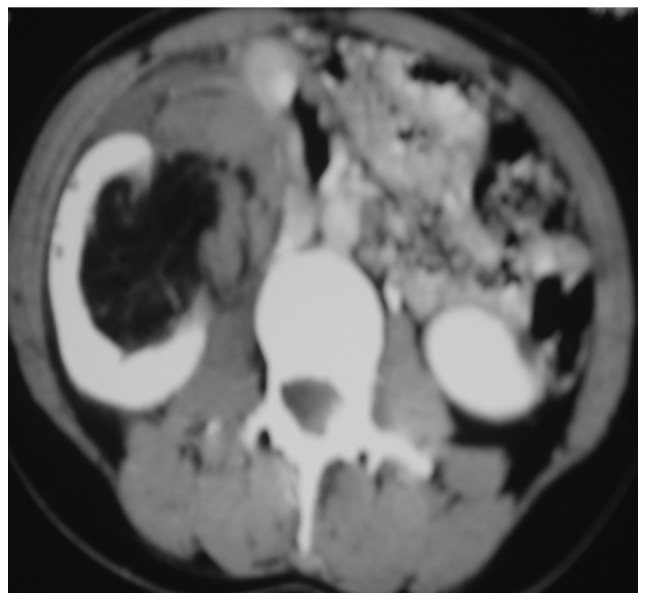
Case 2. Computed tomography (CT) scan of the kidney, demonstrating an oval and low-attenuation mass situated in the right renal sinus. The mass measured ~8×6×8 cm and had a CT value of −70 HU. Contrast-enhanced CT scan showed an enhanced heterogeneous mass in the right kidney.
